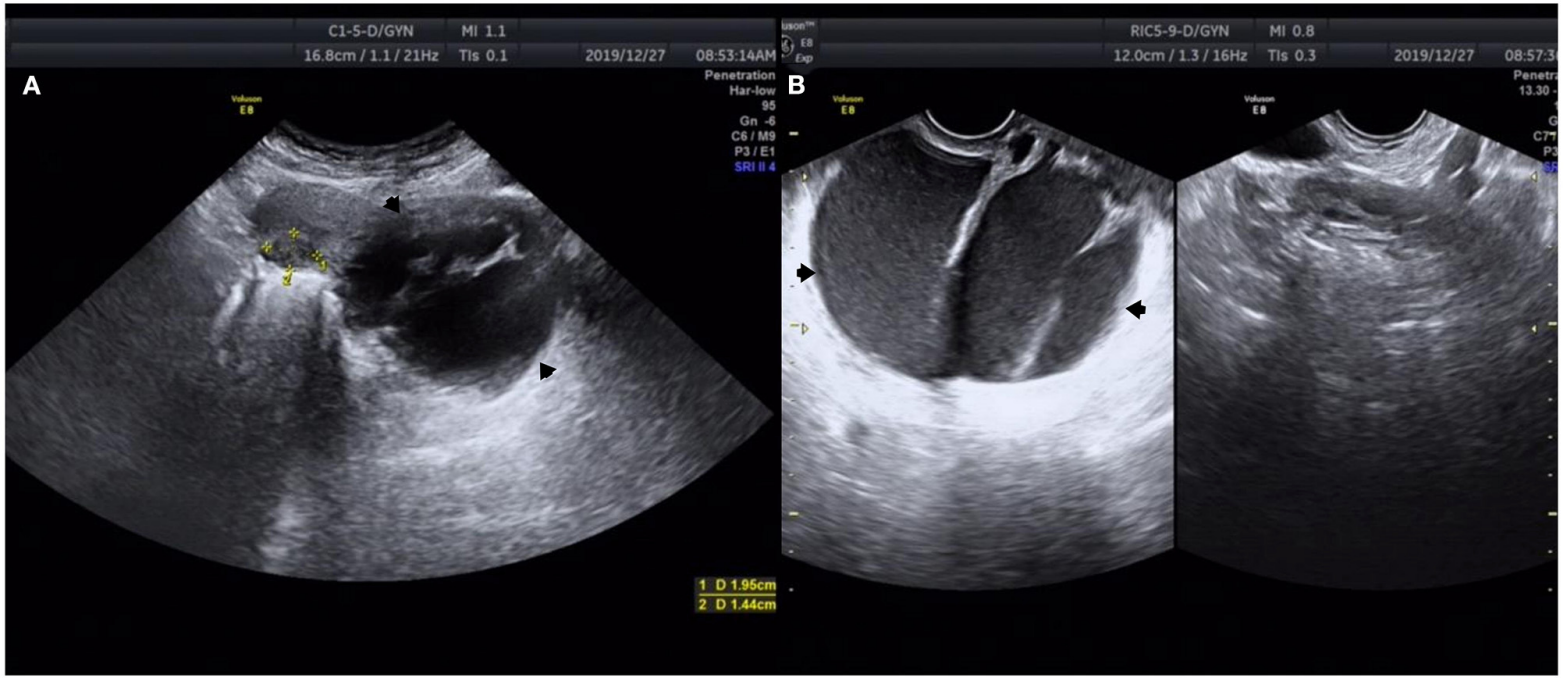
Ultrasound images of a 37-year-old woman with cervical adenocarcinoma. (A) Transabdominal ultrasound shows a 95 × 80 mm cyst below the back of the uterine cervix (black arrow). (B) Transvaginal ultrasound shows that the cyst fluid was viscous and there was a large separation in the cyst (black arrow). *Measurement of uterine leiomyoma markers.
